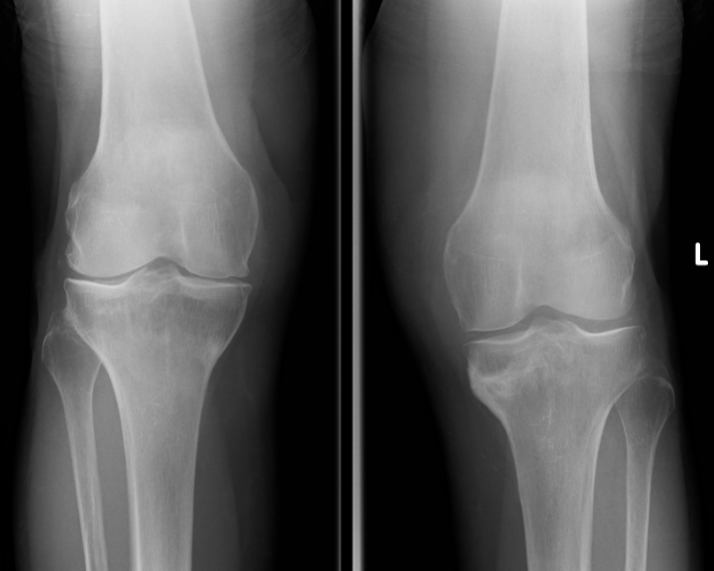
Anteroposterior radiographs seven months after the initial symptoms, showing osteosclerotic change with a periosteal reaction at both medial proximal tibias.
